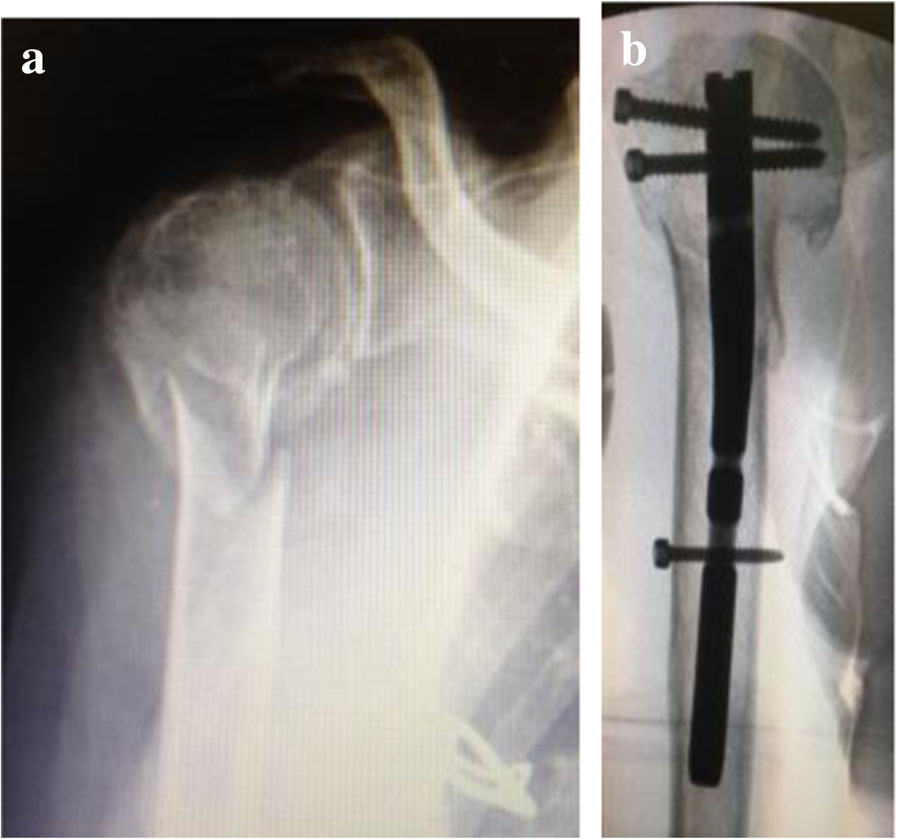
Radiographies of the right shoulder (a) of a 68-year patient with fracture in two parts of the humerus proximal region (Neer classification) and treated with short-locked intramedulary nailing (b), with implant placement through the rotator cuff
